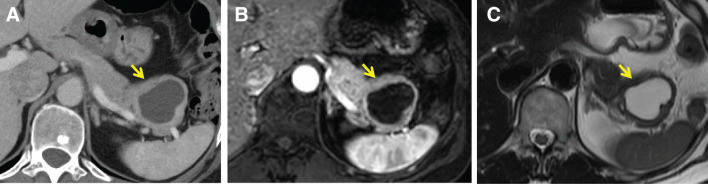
Imaging manifestations of the pancreatic lesions (as indicated by the yellow arrow) in Case 2. (A) CT shows the tail of the pancreas and the cyst wall. (B) MRI reveals a cystic lesion with low signal intensity on T1-weighted images. (C) MRI reveals a cystic lesion with high signal intensity on T2-weighted images.
